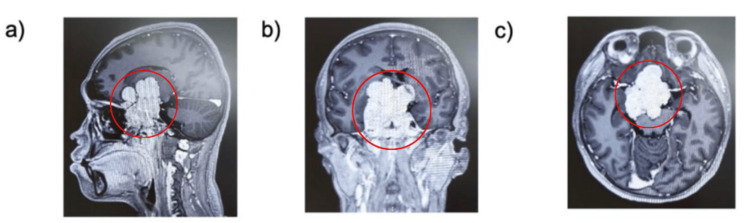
MRI demonstrating a giant invasive pituitary macroadenoma. Sagittal (a), coronal (b), and axial (c) T1-weighted post-contrast images reveal a large sellar and suprasellar mass with heterogeneous enhancement (circled in red). The tumor invades both cavernous sinuses, compresses the optic chiasm, and extends into adjacent structures
